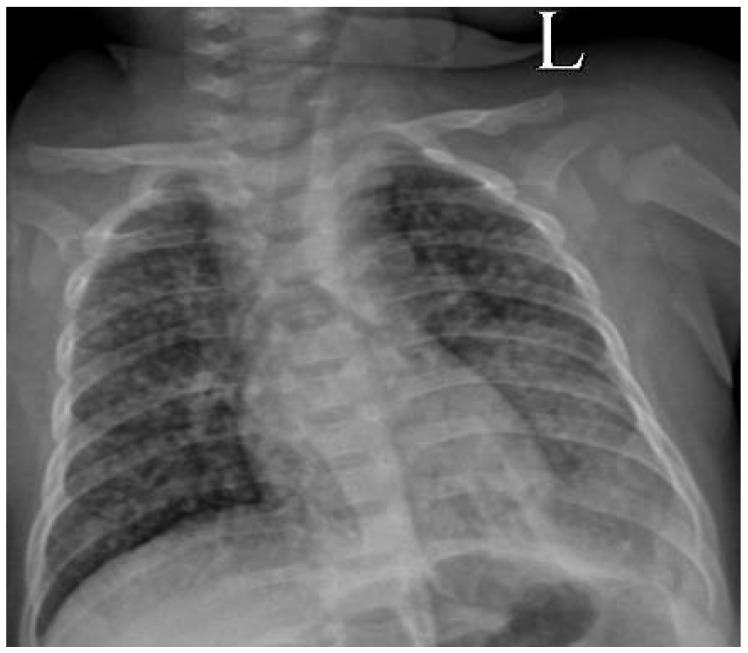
Chest radiograph on admission demonstrating bilateral nodular infiltrates (together with left lower lobe consolidation), suggestive of disseminated (miliary) tuberculosis.
